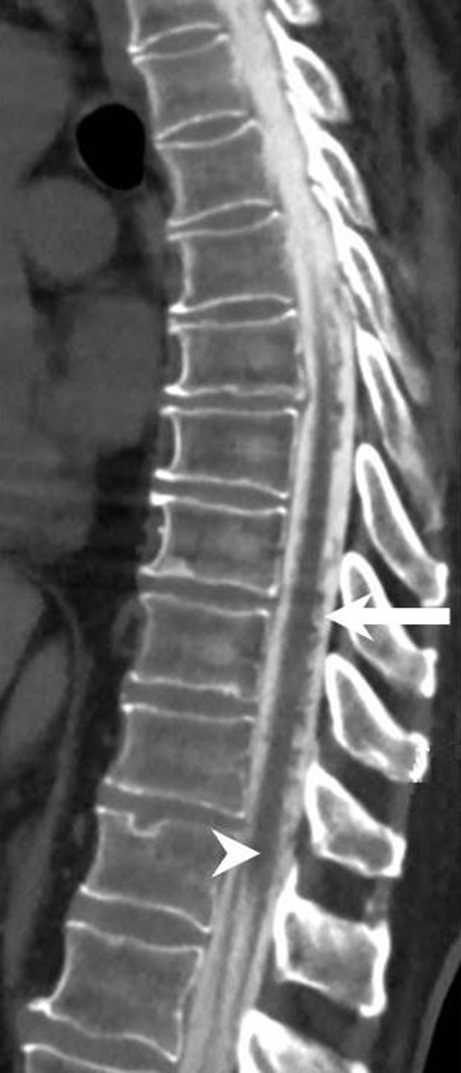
On sagittal reconstruction of a spinal CT after intrathecal administration of contrast agent a dilation of perimedullary veins (*arrow*) along the dorsal surface of the myelon through to the conus (*arrowhead*) is present
